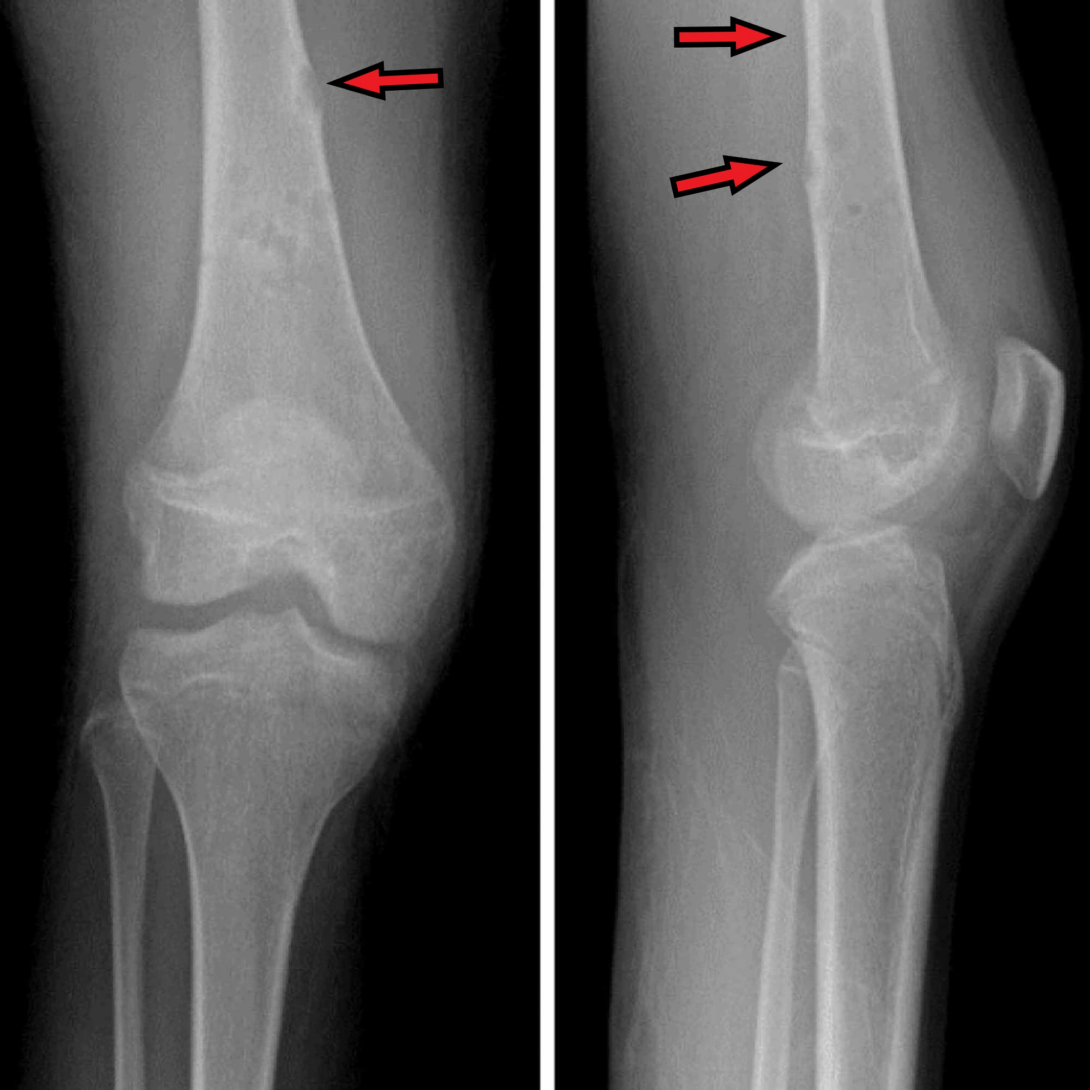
X-ray of the right knee two views (anteroposterior and lateral view) Red arrows showing lytic lesions of the distal femoral metadiaphysis with surrounding reactive sclerosis
